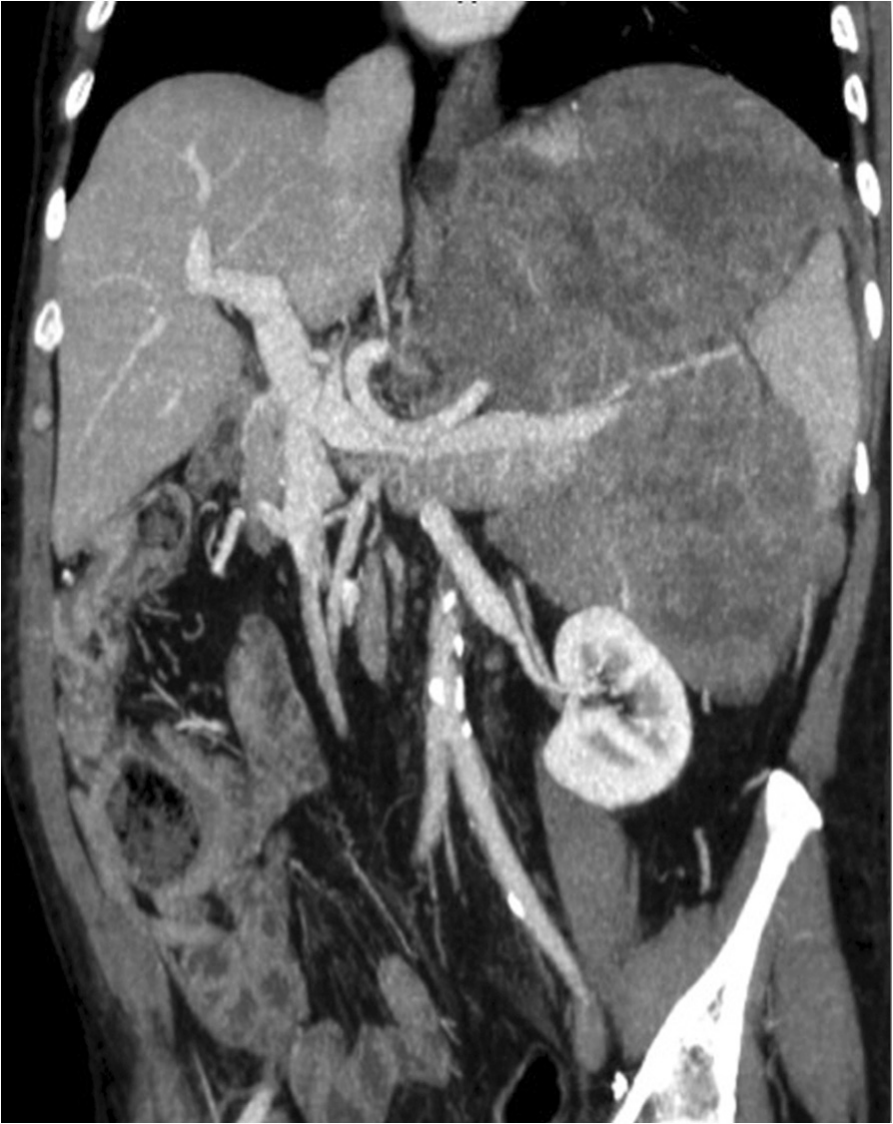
Coronal computed tomogram, showing a large mass of 40 × 25 cm, which extended from the posterior wall of the stomach to the spleen, enveloping the body and the tail of the pancreas.
